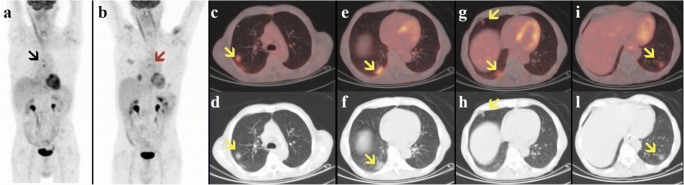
Previous (a) and current (b–l) [18F]FDG PET/CT of an asymptomatic 80-year-old man with a history of non-Hodgkin lymphoma. Maximum intensity projection (a–b), transaxial fused PET/CT (c, e, g, i), and CT (d, f, h, l) images of [18F]FDG uptake in the lungs
